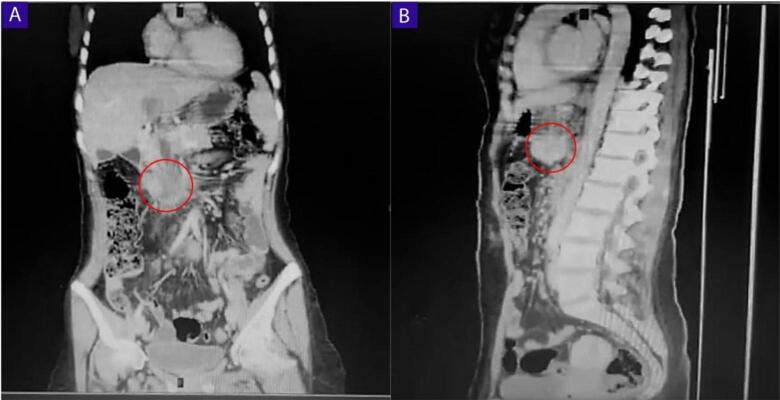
A Abdominal CT scan, coronal section, the cyst can be seen adjacent to the lower vena cava. B Abdominal CT scan, sagittal section, The cyst can be seen adjacent to the lower vena cava.
